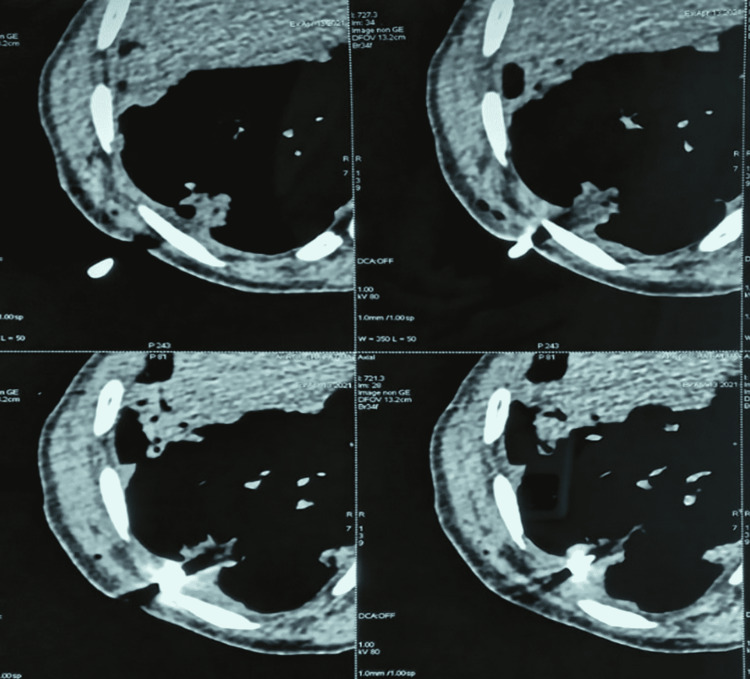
Chest CT scan, mediastinal window: the site of the scan-guided lung biopsy
